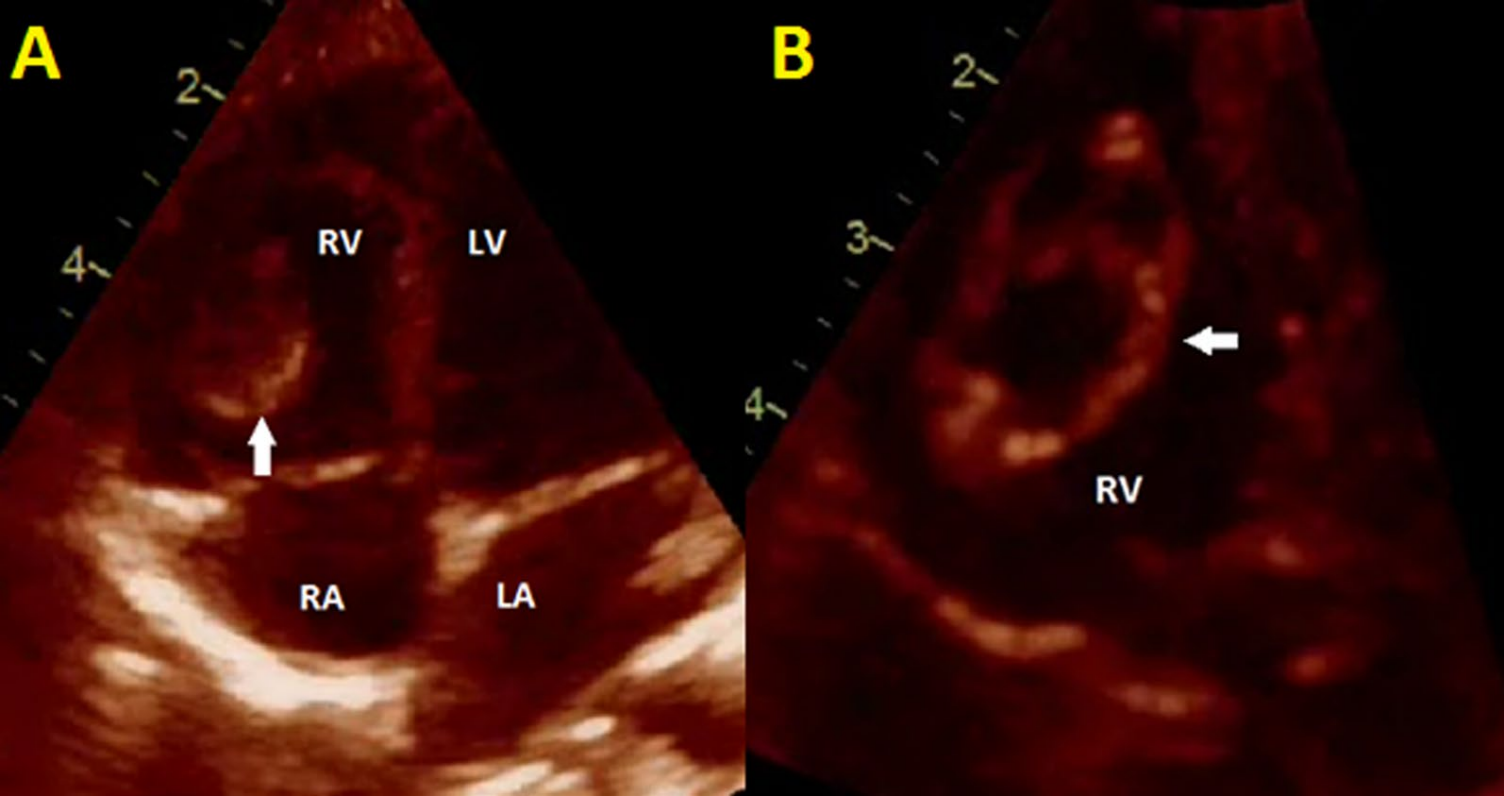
Trans-thoracic echocardiogram at four chamber view A shows large cystic lesion (white Arrows) with adhesion to the right ventricular free wall. Tilting the probe into posterior B reveals a large multivesicle cyst in the right ventricle. *RA* Right atrium; *RV* Right ventricle; *LA* Left atrium; *LV* Left ventricle
